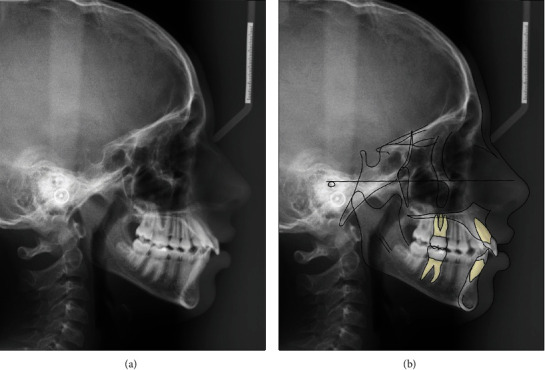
(a) Cephalometric radiograph (b) and cephalometric tracing before treatment.
